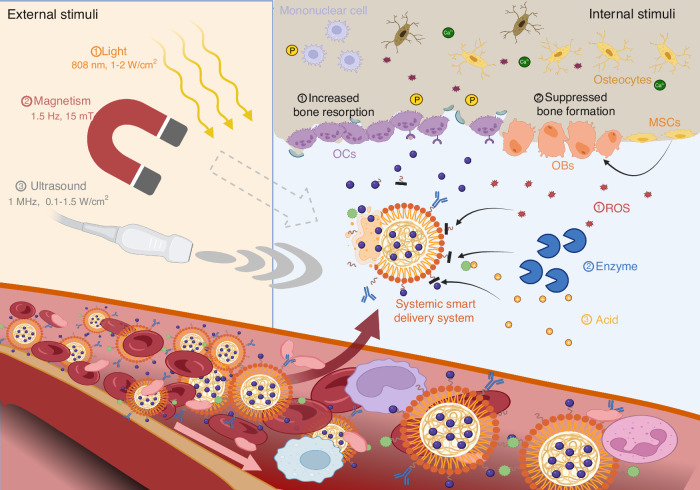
The internal and/or external stimuli activate the systemic smart delivery system to release drugs contributing to bone repair. The systemic smart delivery system patrols the circulation and moves into the degenerated bone microenvironment. The internal stimuli derived from pathophysiological bone tissue include accumulated ROS, specific cleavage enzymes, and an acidic environment, which activate the systemic smart delivery systems. Together with them or alone, the external stimuli, including light, ultrasound, mechanical force, and magnetism, elicit the conformation change and phase transition of the delivery system to release drugs for bone repair and regeneration. OBs: osteoblasts, MSCs: mesenchymal stem cells, OCs: osteoclasts. Created by BioRender software (biorender.com)
